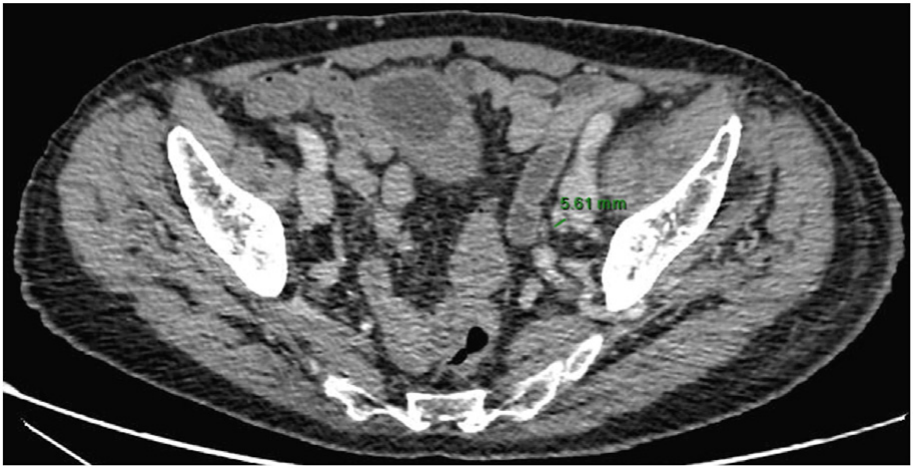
Axial computed tomography scan: regression of a left external iliac lymph node (13.69 mm to 5.61 mm from February to August).
